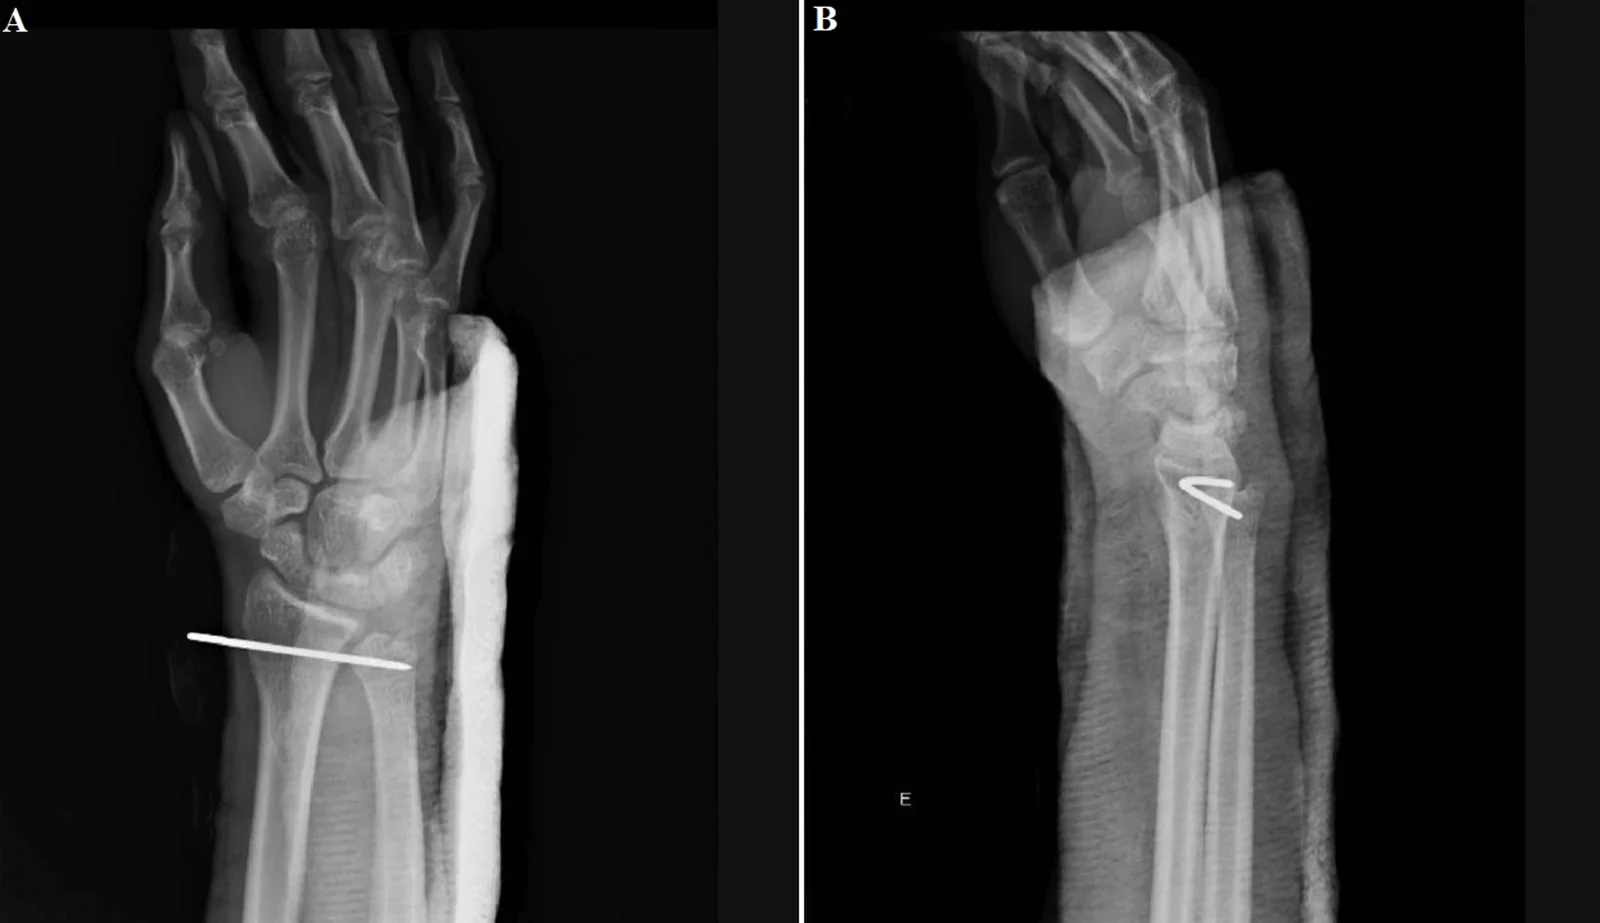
Follow-up radiographs 20 days after surgery showing maintained DRUJ reduction with percutaneous fixation and immobilization. (A) Anteroposterior view. (B) Lateral view. DRUJ: distal radioulnar joint
